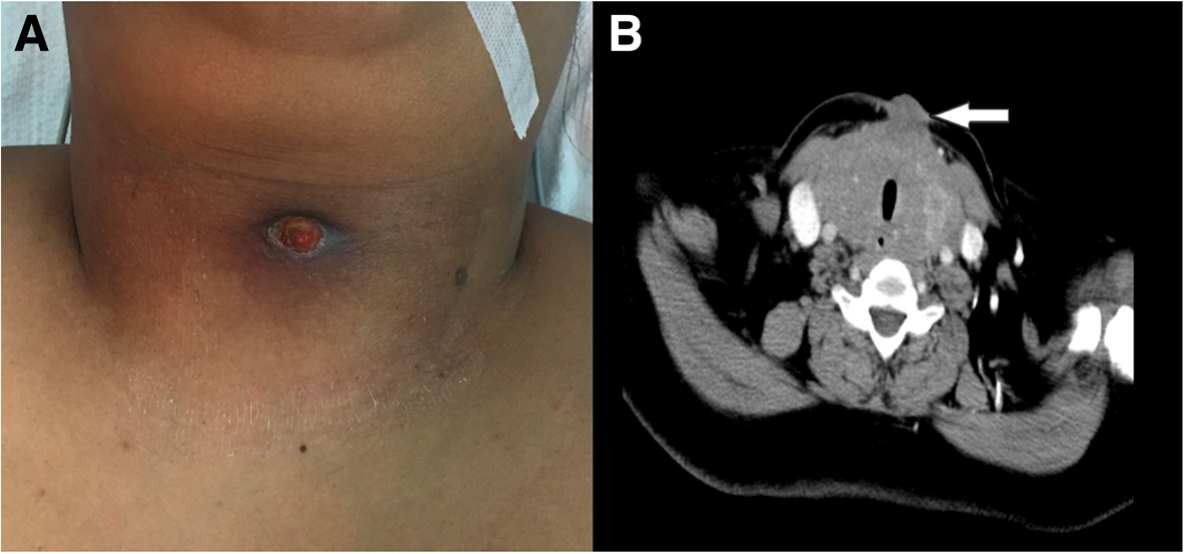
Anterior midline neck sinus secondary to infiltrating thyroid cancer (a); CT scan transverse view: diffuse enlargement of thyroid gland infiltrating the skin with heterogeneous density (b)
